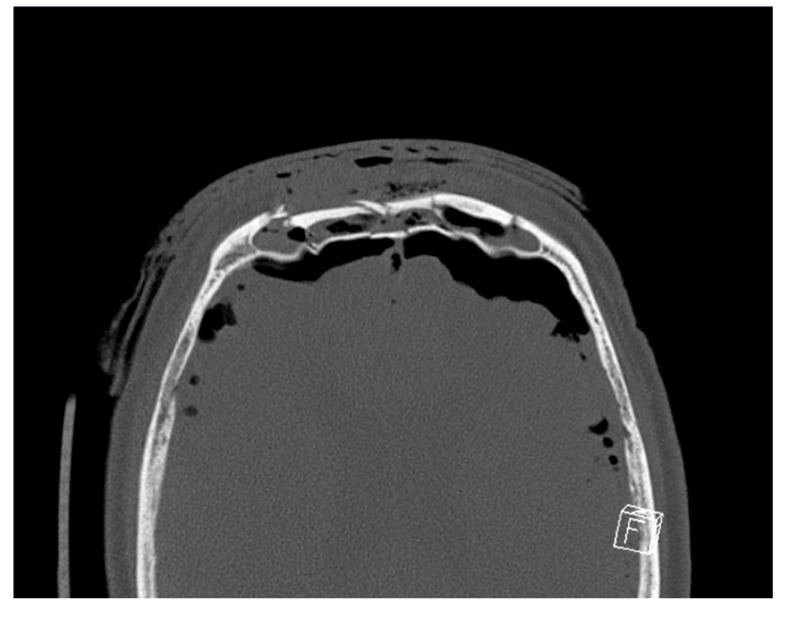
Axial CT section of a patient showing comminuted outer and inner tables and large volume pneumocephalus.
